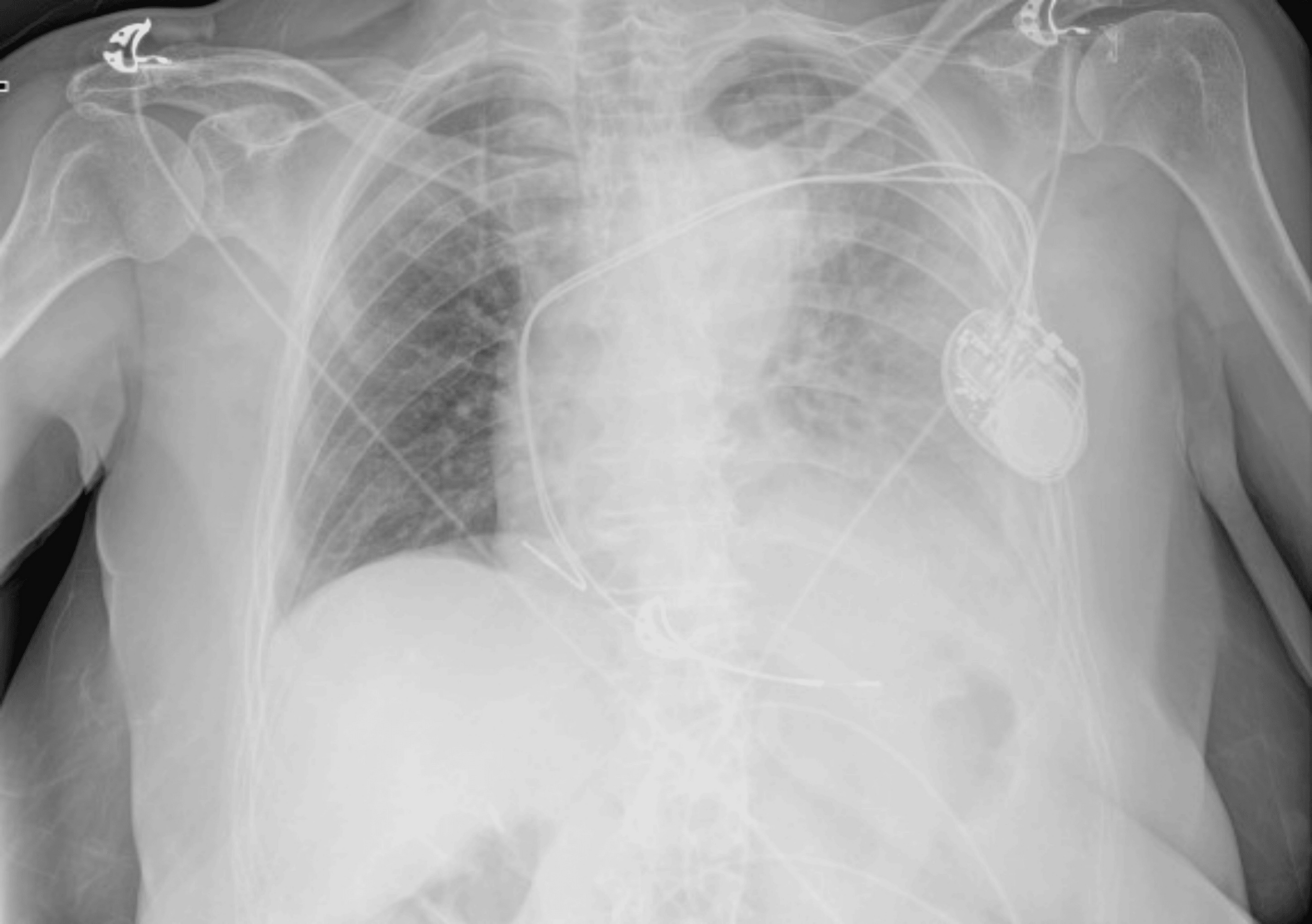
Chest X-ray performed after implantation Correctly placed pacemaker leads with new-onset left pleural effusion.
